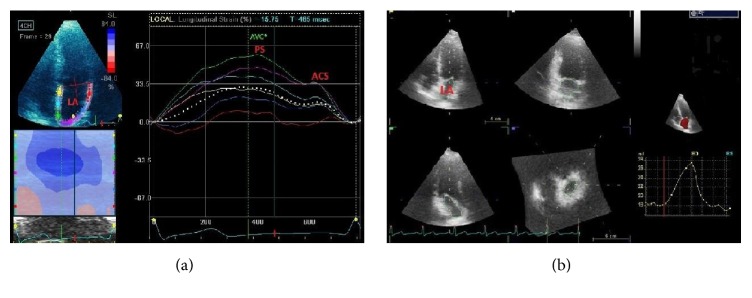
Left atrial (LA) size and function in systemic arterial hypertension. Novel technologies for LA analysis. (a) LA wall deformation curve by speckle tracking echocardiography. (b) LA volume by three-dimensional echocardiography. ACS = atrial contraction strain; PS = peak strain (peak global longitudinal strain at ventricular end-systole).
